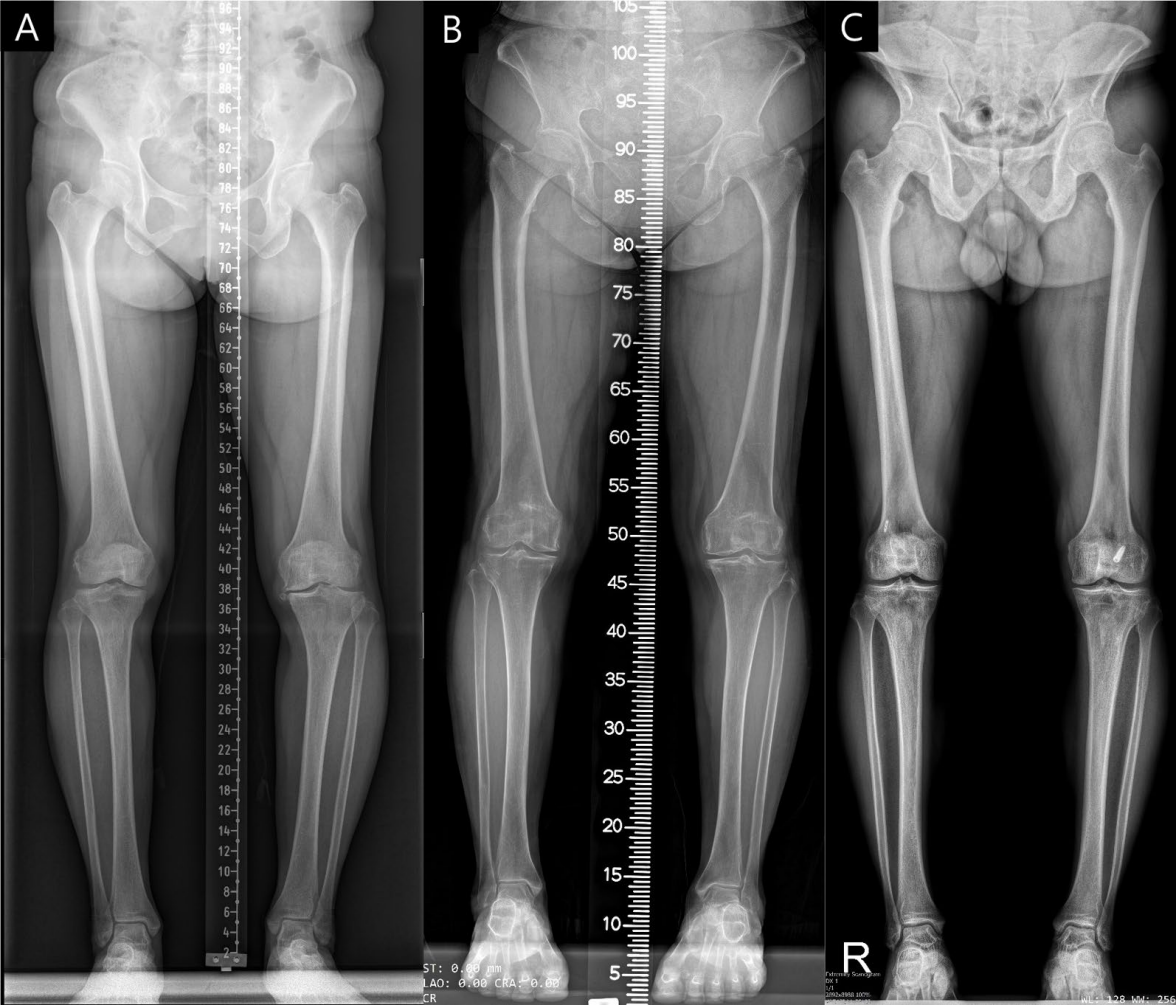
Examples of full-leg radiographs from Institutes A, B, and C, with each letter representing the corresponding institute
